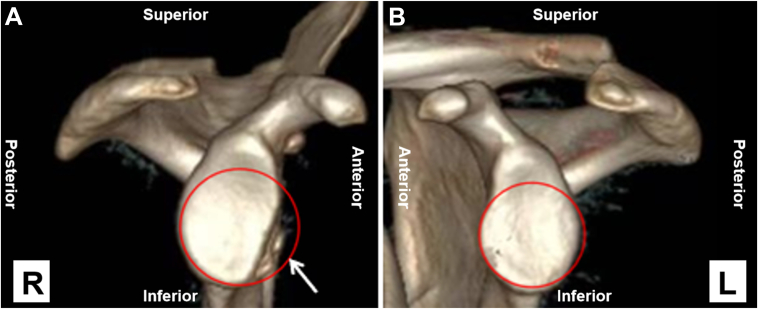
Preoperative 3-dimensional computed tomography scans of the patient's right shoulder, demonstrating the glenoid defect (A), and the normal left shoulder (B). Significant anterior glenoid bone loss is present in the right shoulder (white arrow).
